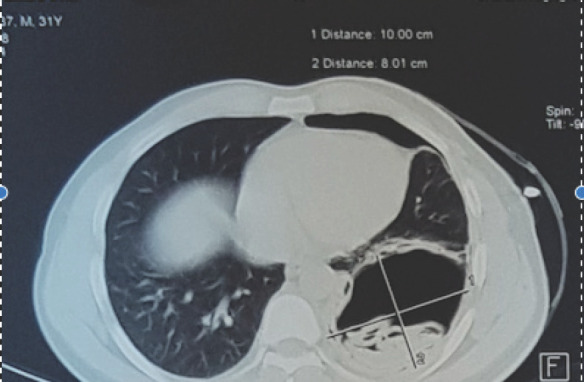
CT chest showing cavitatory lesion with laminated membrane within the cavity.
